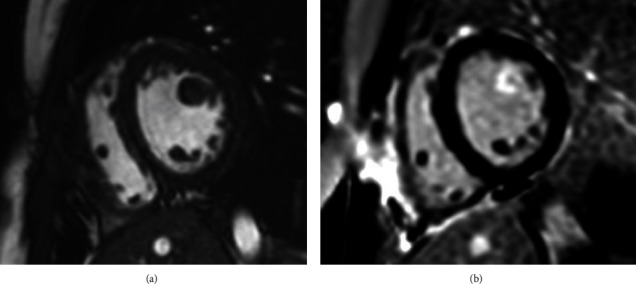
Cardiac MRI—short-axis views. (a) Cine acquisition: note the hypertrophy of the anterolateral papillary muscle; (b) phase-sensitive inversion recovery (PSIR) sequence: late gadolinium enhancement (LGE) of the anterolateral papillary muscle.
